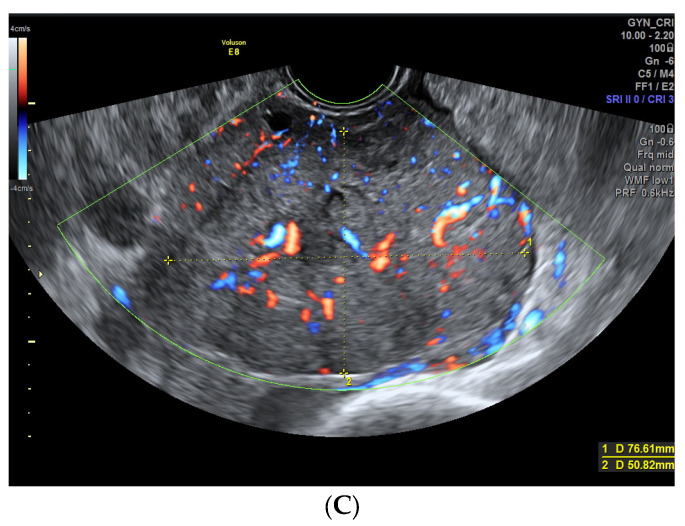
(A) Fibroma in a 59-year-old woman. A solid regular mass with smooth contour can be seen with scarce Doppler color (score color 2). (B,C) Malignant ovarian lesions with irregular contour are shown. Image (B) shows a cystic mass with an irregular internal contour corresponding to a 55-year-old woman with a clear cell carcinoma. Image (C) shows a solid mass with moderate-intense Doppler color (score 3–4) with an irregular external contour, corresponding to a serous ovarian carcinoma in a 64-year-old woman.
